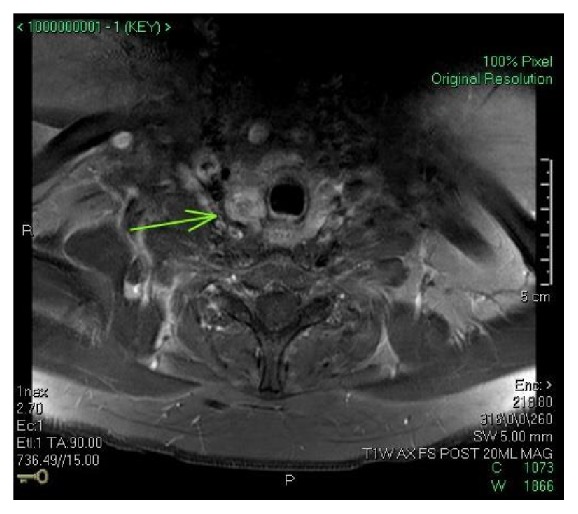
Axial section from the preoperative magnetic resonance images showing the same mass.
